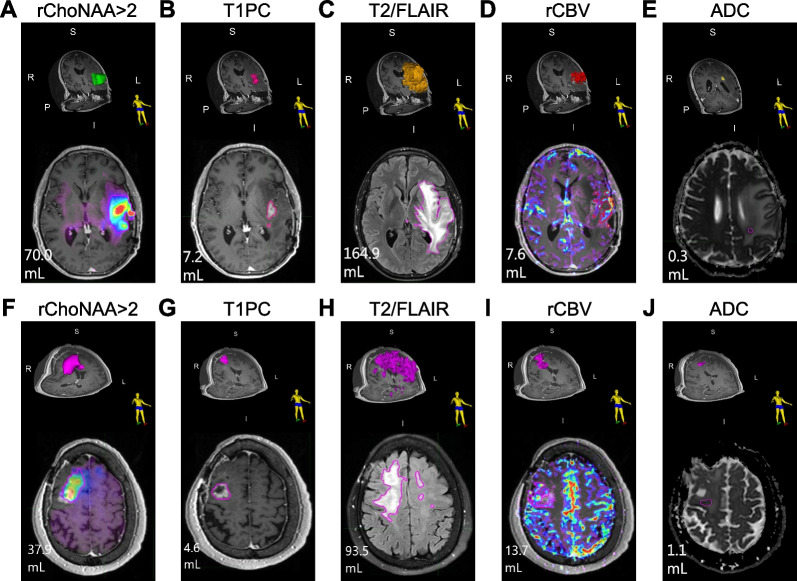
Example patients in selected axial slice and representative 3-dimensional volumetric contour. A–E. Example contours for patient with rGBM show rChoNAA > 2 volume, T1PC volume, T2/FLAIR volume, rCBV volume, and ADC volume. Pink contours indicate the areas of rChoNAA > 2, contrast enhancement, FLAIR hyperintensity, rCBV elevation, and diffusion restriction. F–J Example contours from a separate patient with rGBM shows rChoNAA > 2 volume, T1PC volume, T2/FLAIR volume, rCBV volume, and ADC volume. Pink contours indicate the areas of rChoNAA > 2, contrast enhancement, FLAIR hyperintensity, rCBV elevation, and diffusion restriction. I: inferior, L: left, P: posterior, R: right, S: superior, mL: milliliter
